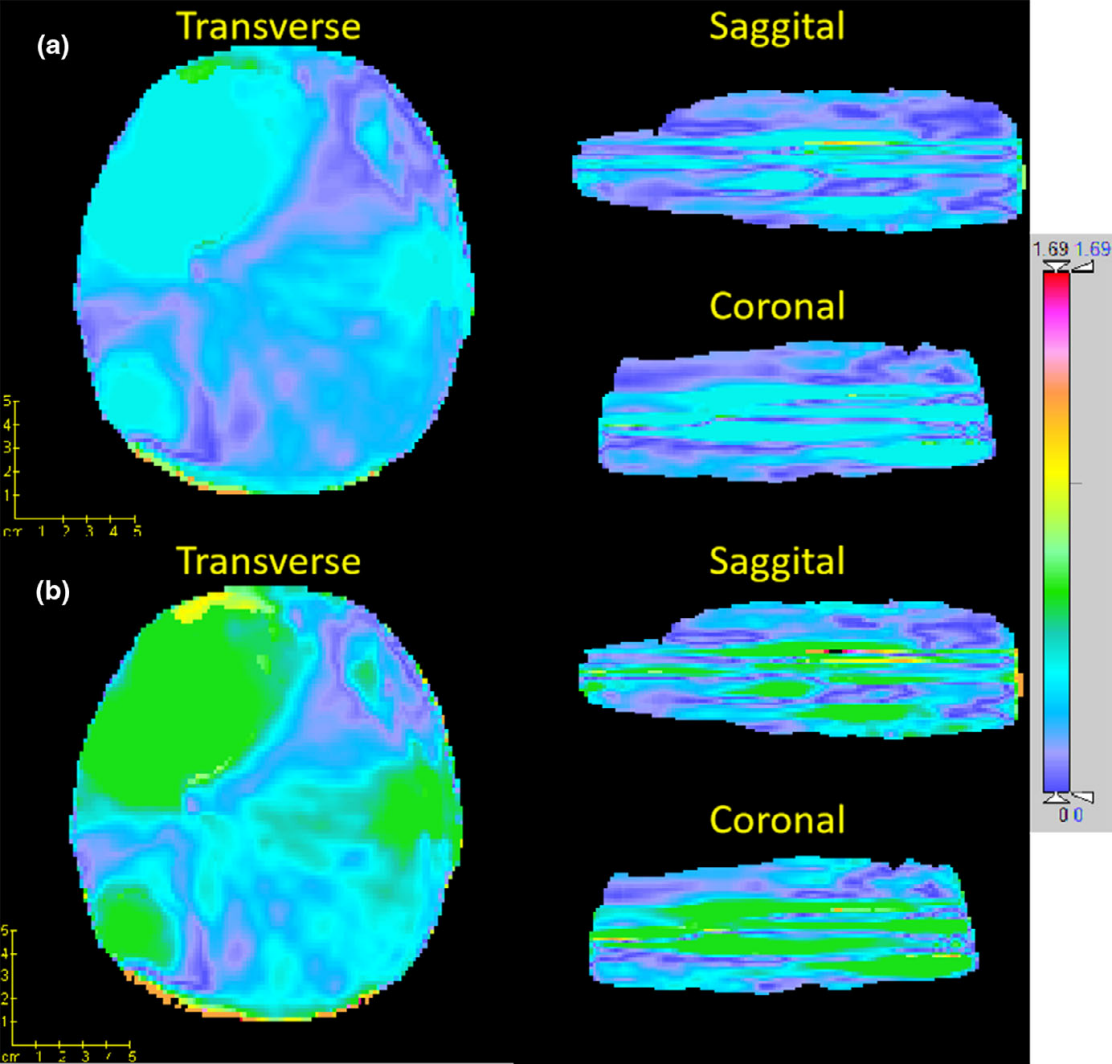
Gamma analysis between the dose distribution of synthetic and planning computed tomography in transverse, sagittal, and coronal planes with criteria of 3 mm/3% (a) and 2 mm/2% (b), respectively.
